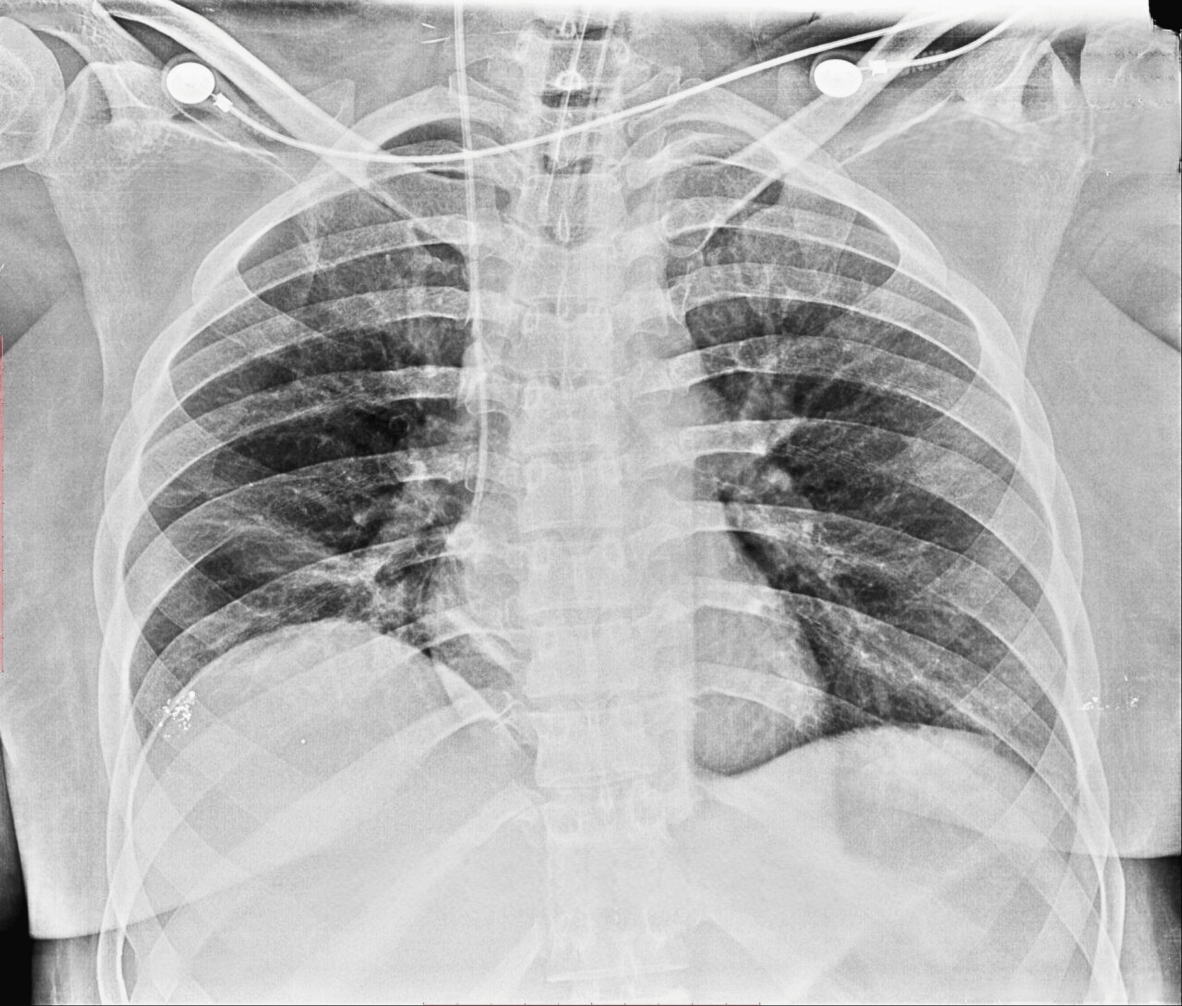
Portable ICU chest X-ray (supine, AP view). The image shows limited visualization of bilateral lung fields. No obvious gross abnormality is observed. ICU: intensive care unit; AP: anteroposterior
